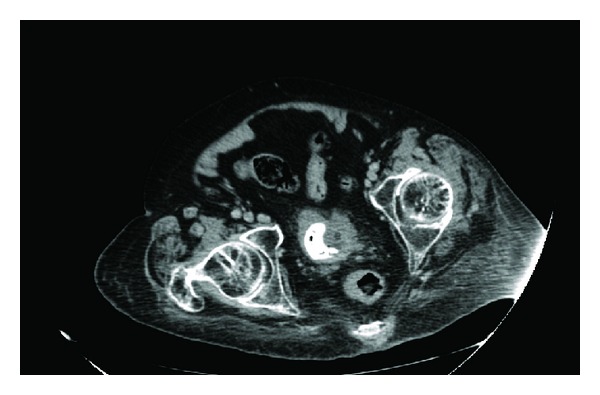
Axial section of computed tomography of abdomen and pelvis: a 31 mm calculus was present in bladder; the stone contained gas in its matrix. Findings of gas within the matrix of vesical calculus indicated that urine infection due to gas forming organisms such as *Escherichia coli* or *Candida albicans* had been present for some time.
